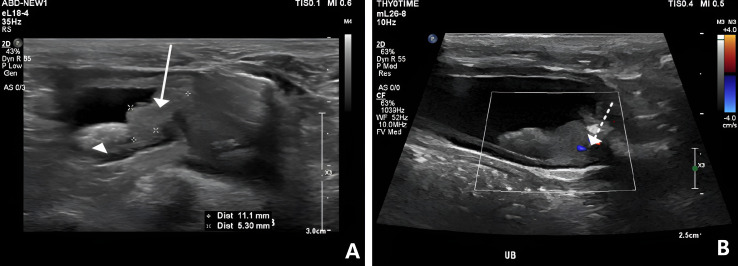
Ultrasonography of a trigonal hyperechoic bladder mass. (A) An irregularly marginated hyperechoic nodule (5.3 × 11.1 mm) (arrow with line) and calculi (arrow head) was observed at the urinary bladder trigone. (B) The mass contained calcification and exhibits clearly detectable vascular flow (arrow with dotted line).
